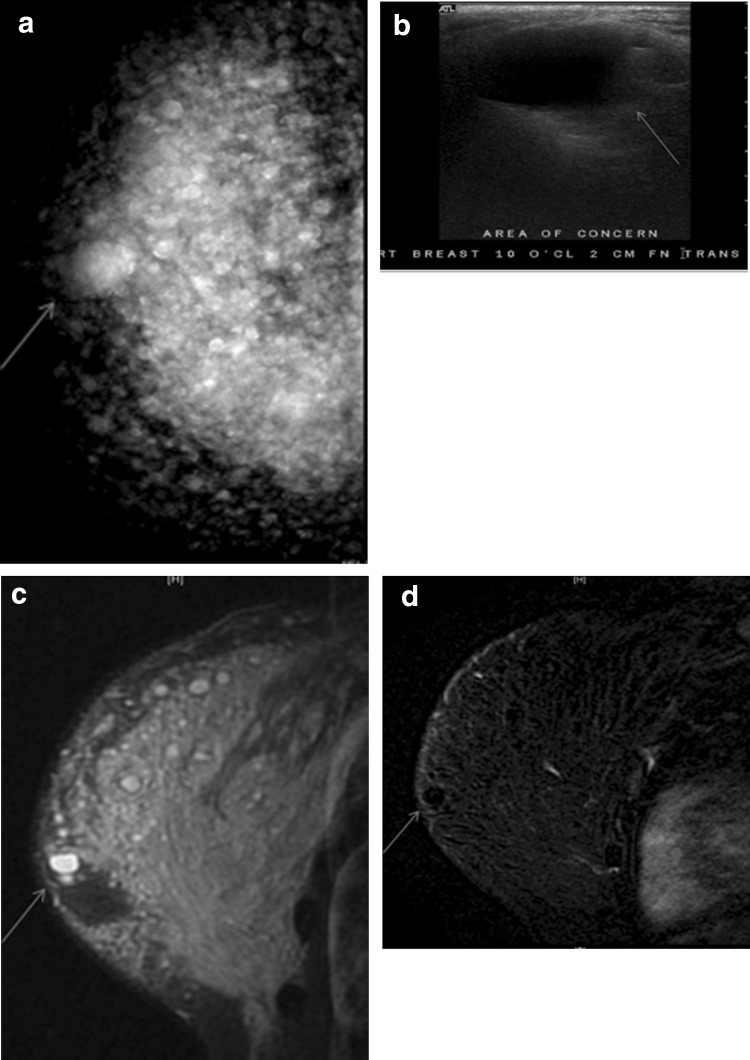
A 60-year-old transgender woman with free silicone injections feels a lump. a Mammogram shows multiple high-density masses which are silicone granulomas. The palpable granuloma is designated by blue arrow. b Ultrasound shows the palpable silicone granuloma is a circumscribed anechoic mass (arrow). c The silicone granuloma is a very high signal circumscribed mass on MRI T2 STIR sequence (arrow). d On a TI post-contrast sequence the silicone granuloma is a non-enhancing circumscribed mass (arrow)
